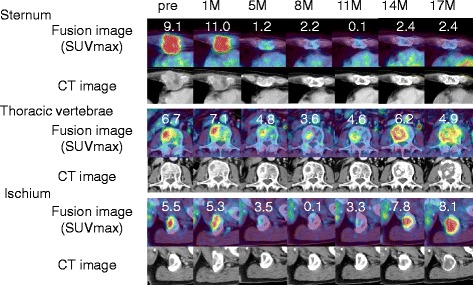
The imaging of a practice case: 70’s-year-old patient with sternum, thoracic vertebrae, and ischium bone metastases (#20). The SUVmax of all bone metastases decreased temporally and increased before tumor was judged as PD. Fusion images were upper lanes. The number means SUVmax of each lesion. CT images were lower lanes. “Pre” refers to pretreatment evaluation; “M” refer to month after TKI treatment started
